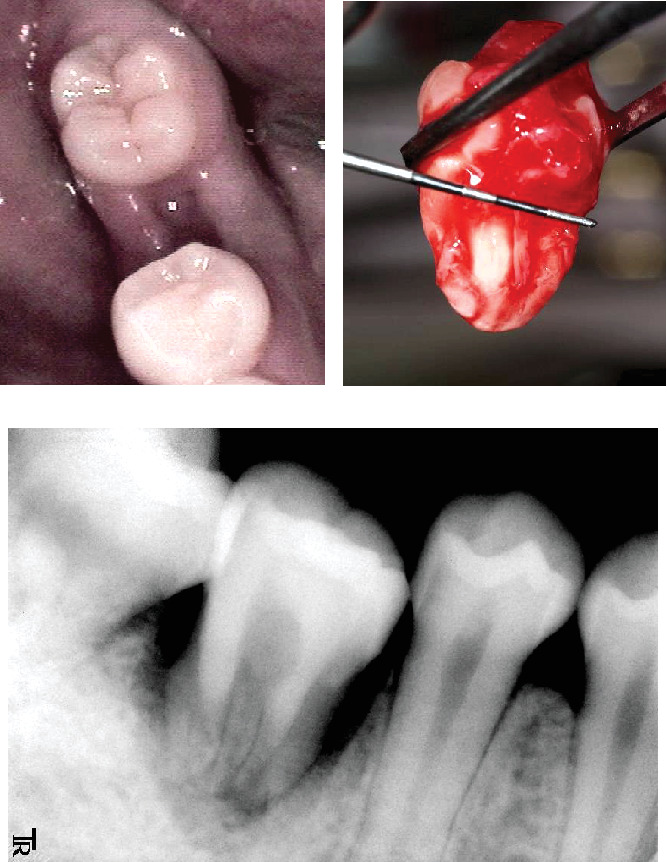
(a) A clinical picture of the recipient site after extraction at the transplantation. (b) The extracted maxillary right third molar for transplantation. (c) A radiograph taken at 2 weeks after the transplantation.
